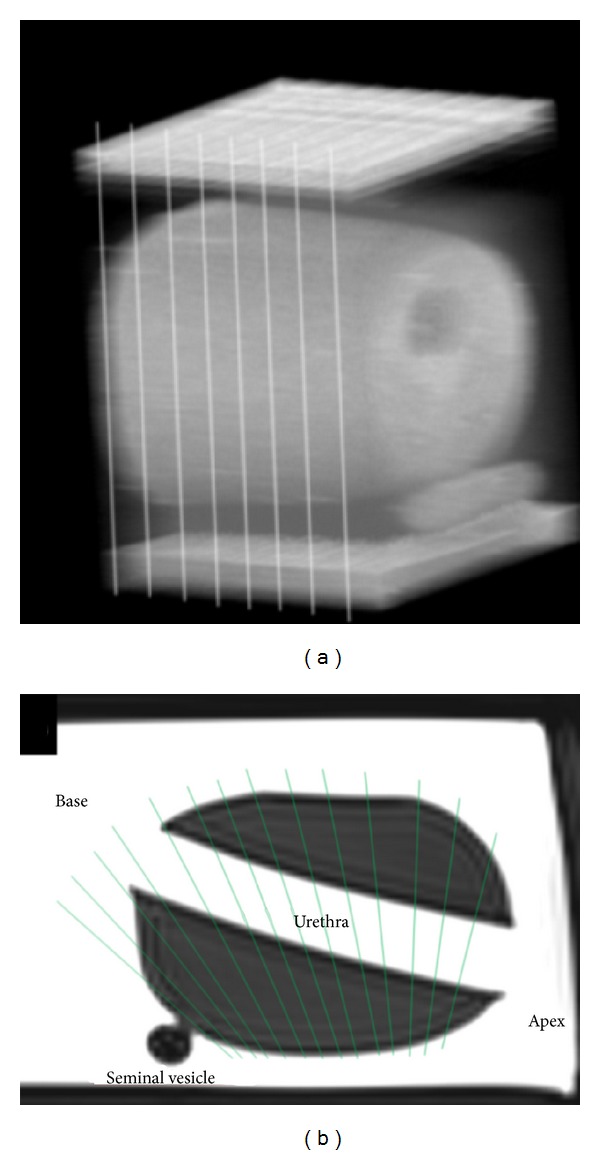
Whole-mount step sections and MRI obtain planes with nearly 90° to the urethra shown in image (a); RTE using an endfire probe obtains planes with different angles in different prostate sectors shown in image (b).
